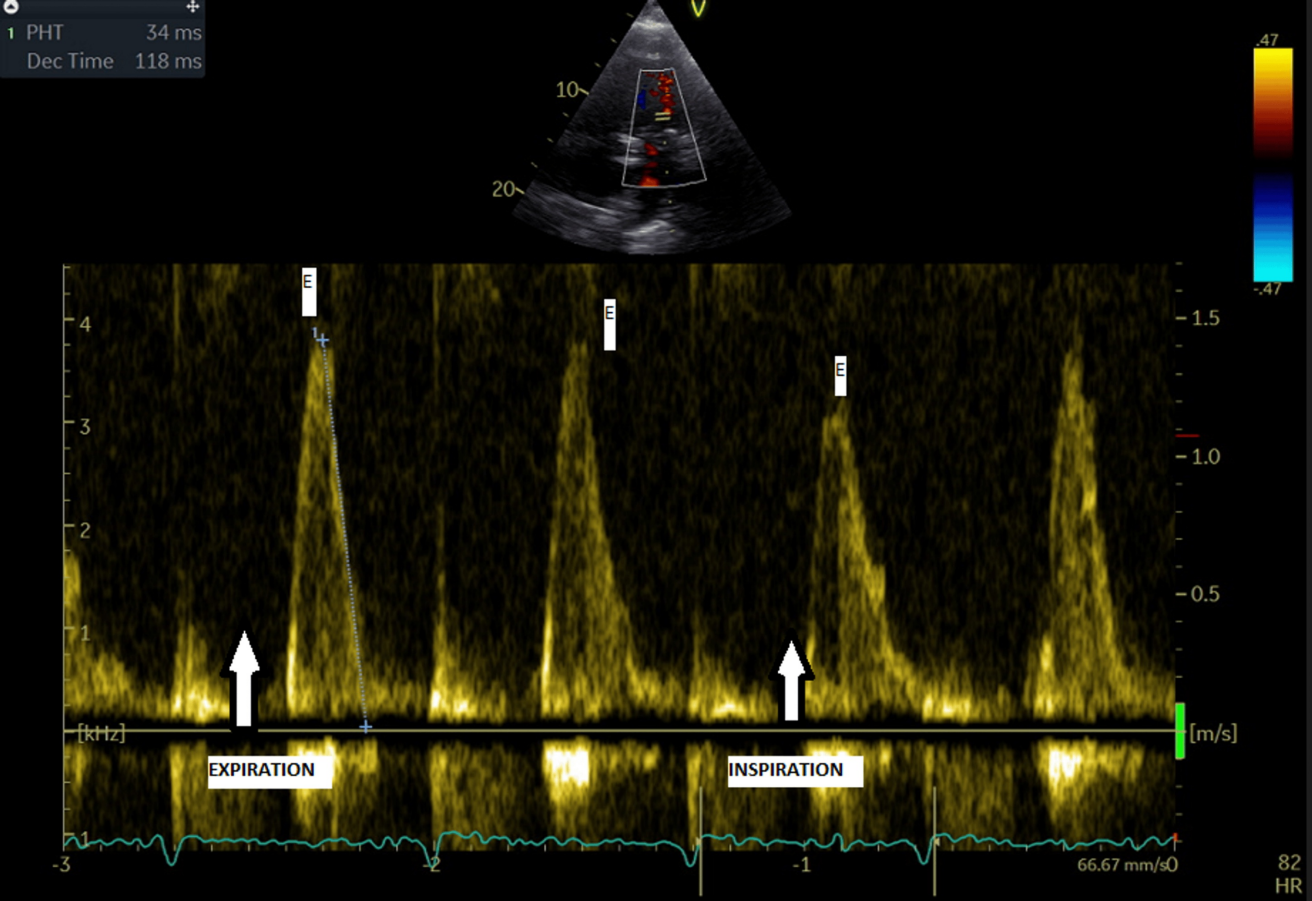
Echocardiography Pulsed Doppler transmitral shows an E wave velocity of approximately 1.5 m/s, with a deceleration time of the E wave of 118 ms, this respiratory variation is a characteristic sign of constrictive pericarditis
